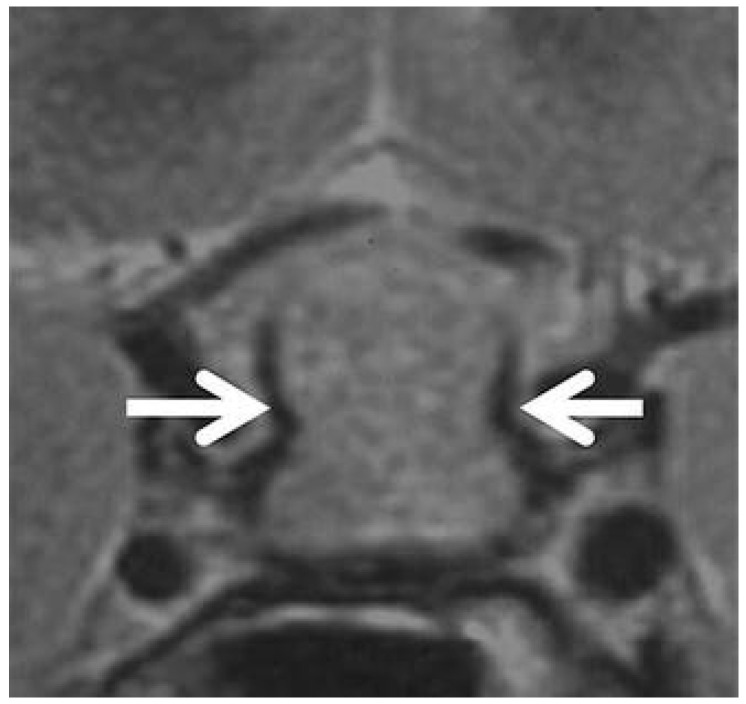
Coronal T2-weighted image showing a peripheral hypointense rim surrounding the parasellar region (white arrows) in a patient with a sellar mass extending superiorly into the suprasellar cistern, consistent with hypophysitis [81].
